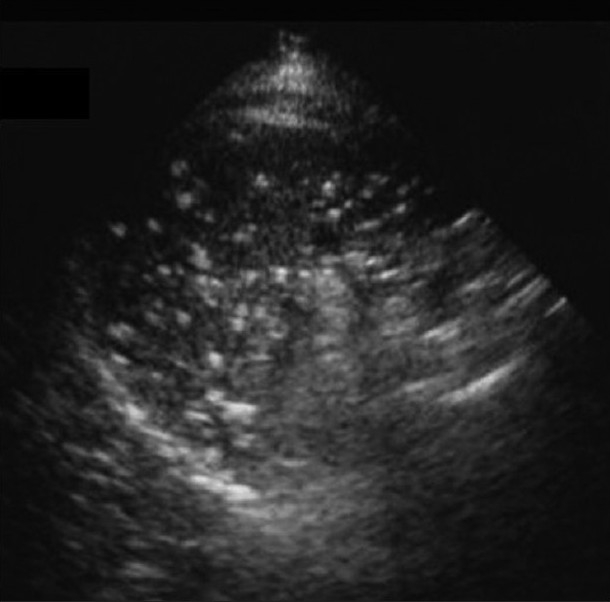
Ultrasound of the spleen on the same patient as in Figure 41 showing multiple microabscesses due to systemic cytomegalovirus infection
